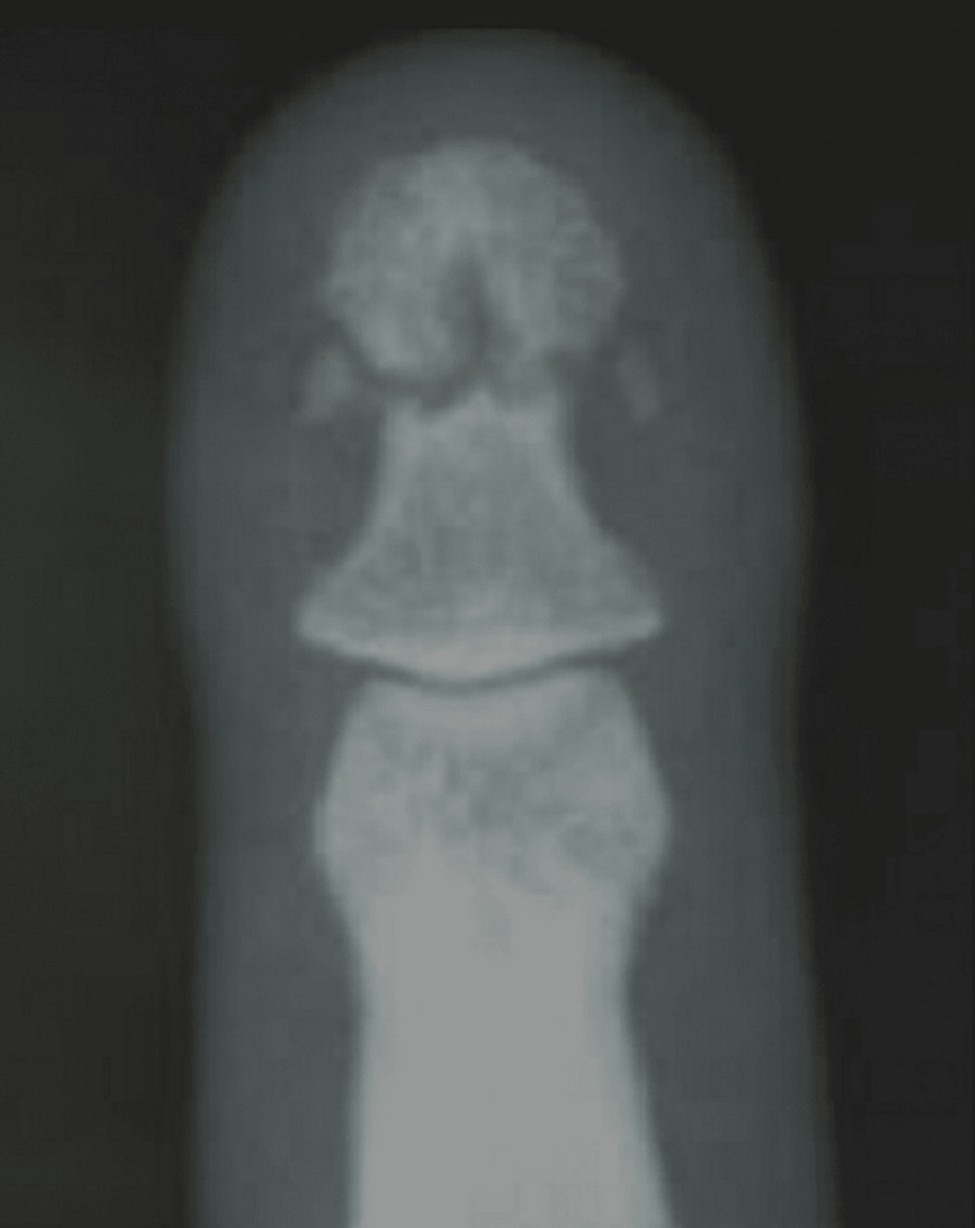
Pre-op X-ray of distal phalanx after reduction of the fracture
